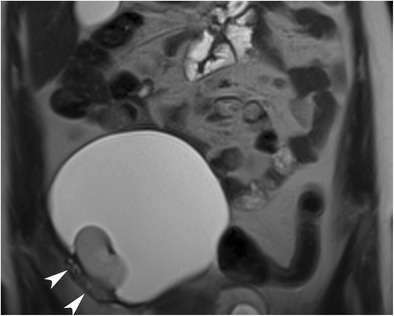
Coronal T2-weighted MR image of a 21-year-old female with mature cystic teratoma. A bean-shaped Rokitansky nodule is seen lying on the right side of the wall of the cystic mass. Ovarian parenchyma with normal signal intensity (arrowheads) can be seen near the cyst wall where the Rokitansky nodule arises
